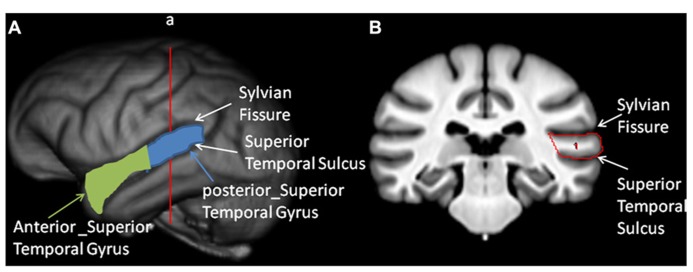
Tracing of the anterior and posterior temporal lobe.  Left panel: (A) 3-D reconstruction of the chimpanzee brain with the sylvian fissure (SF) and superior temporal sulcus (STS) labeled. Also, in green and light blue colors, the anterior and posterior superior temporal gyrus are outlined. Right panel: (B) Coronal view of the sulcl landmarks used to trace the superior temporal gyrus on a T-1 weighted MRI scan.
